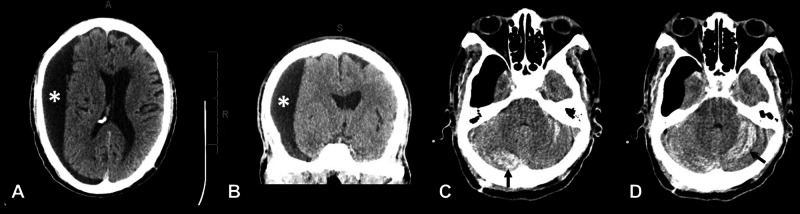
Case of bilateral RCH following burr hole evacuation of a right cystic hygroma. (A & B) Large right holohemispheric subdural hygroma measuring 2.8 cm in widest diameter with right hemispheric sulcal effacement and 10 mm of leftward midline shift. (C & D) s/p right-sided burr holes and placement of a subdural drain noted on postop CT were new bilateral cerebellar hemorrhages. *Right subdural cystic hygroma. RCH: remote cerebellar hemorrhage
